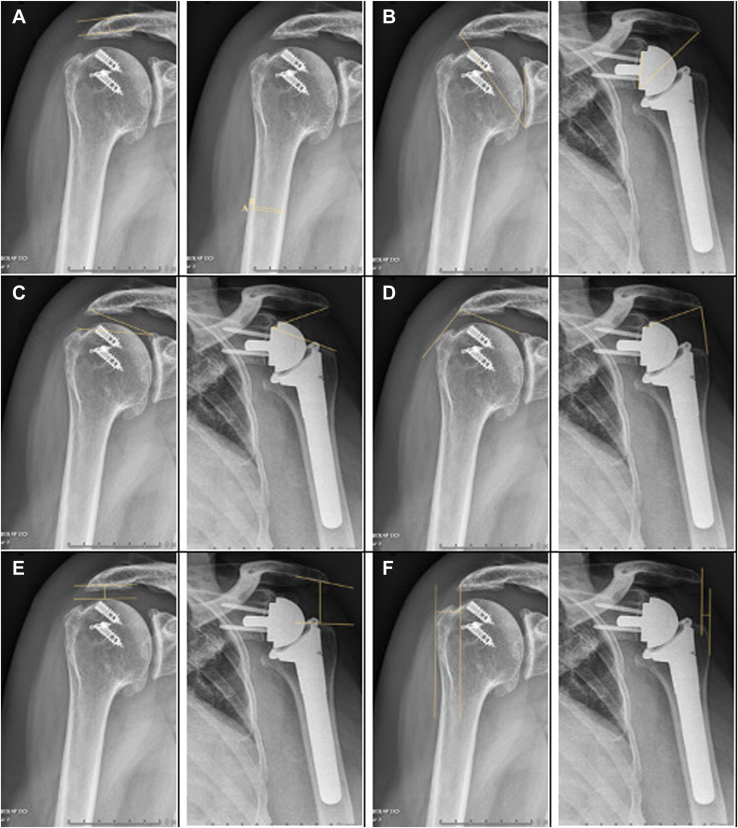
This figure is a visualization of the preoperative and post-operative radiographic parameters on a standard Anteroposterior view of the shoulder. (A) Acromial thickness (AT) and deltoid tuberosity index (DTI); (B) critical shoulder angle (CSA); (C) distalization shoulder angle (DSA); (D) lateralization shoulder angle (LSA); (E) acromiohumeral distance (ACHD); (F) distance from the lateral acromion to the most lateral acromion-to-greater tubercle distance (LAGT).
